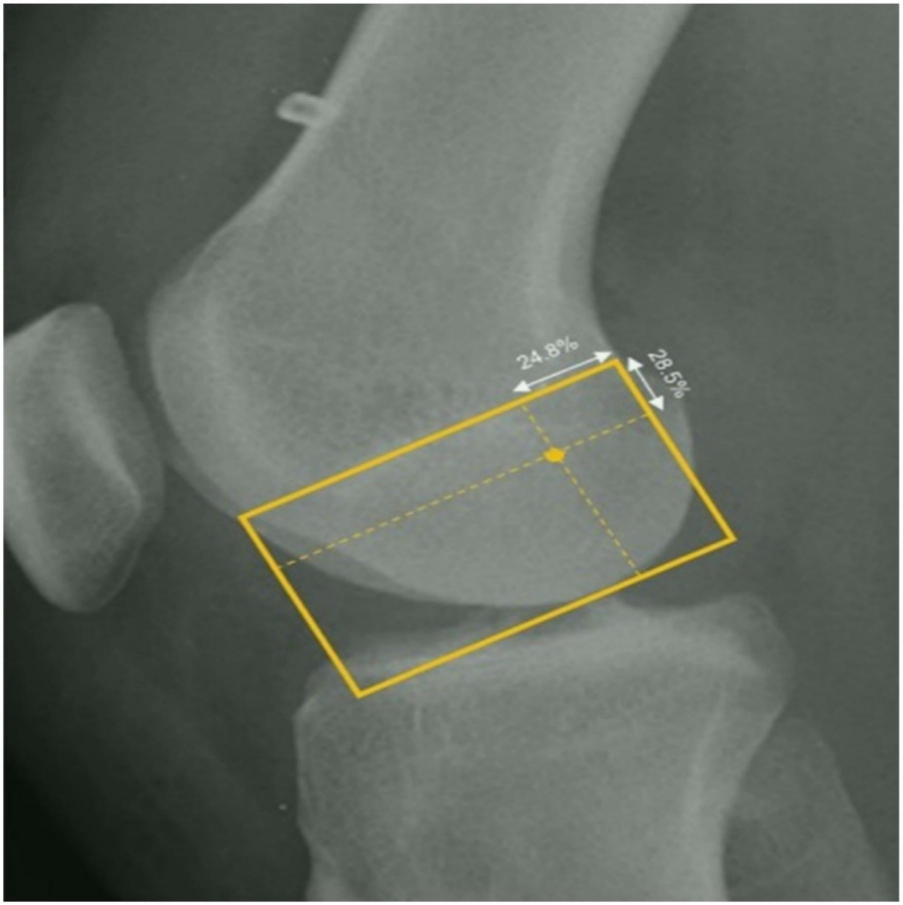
Lateral radiograph of knee with measurement of femoral tunnel positioning using the method by bernard (10).
